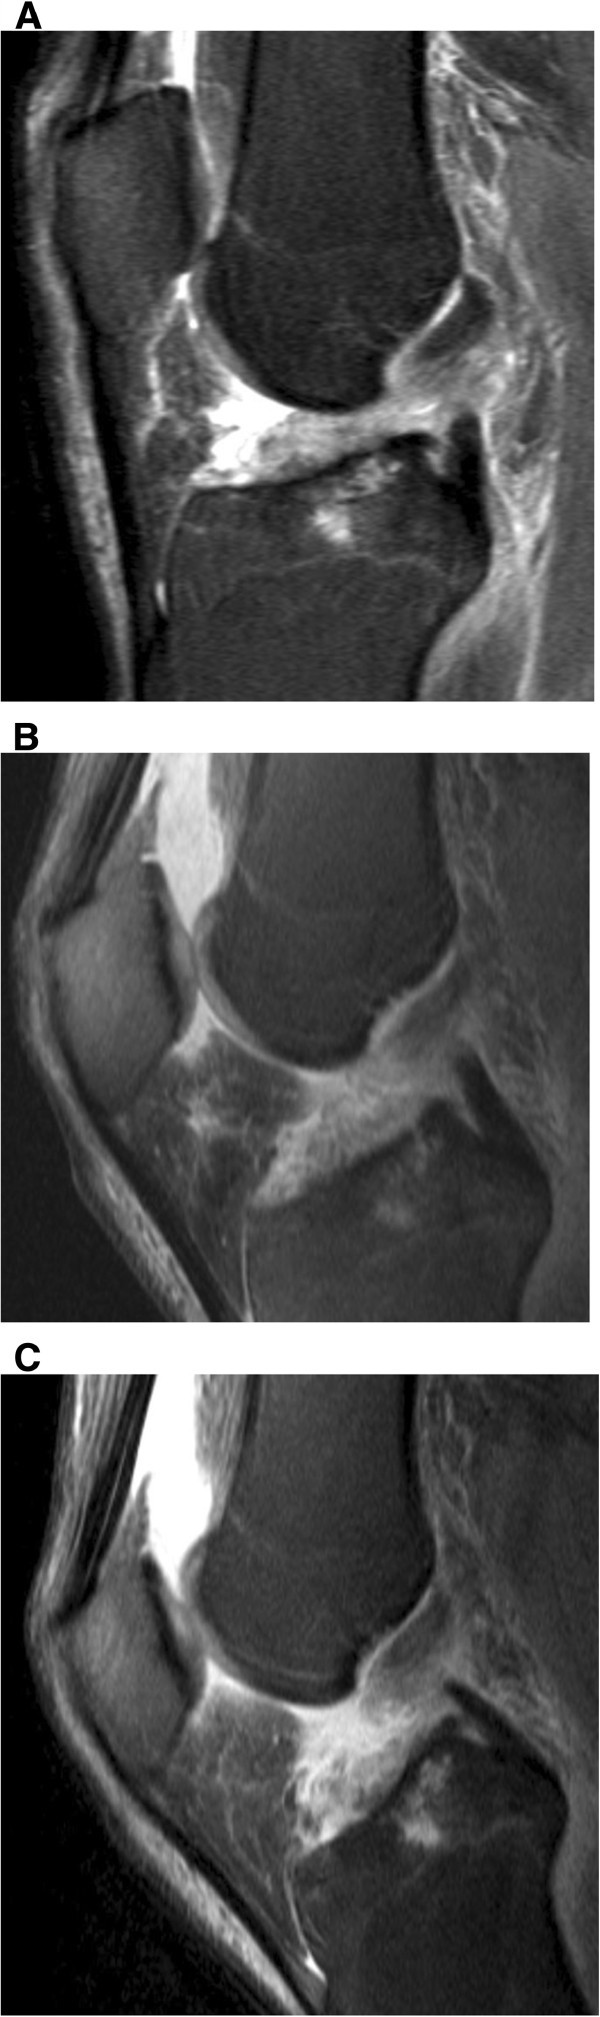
Complete ACL rupture in a 50-year old patient. T2-weighted TSE FS sagittal image (2900/90) obtained at extension (A), 30° (B), and 55° (C) of knee flexion better demonstrates the complete disruption and retraction of the torn ACL fibers than MR images taken at knee extension (A). At knee extension an accurate differentiation between a partial and complete ACL rupture is not possible.
